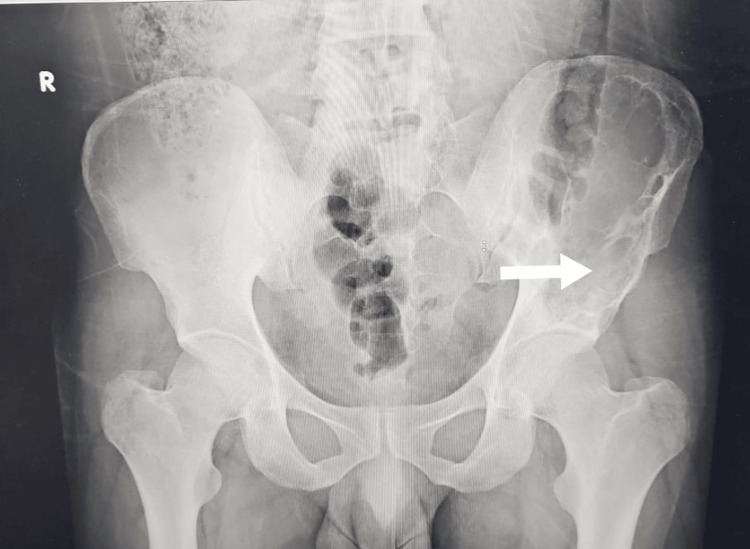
Follow up plain X-ray of pelvis shows the improvement of bone quality in the left iliac bone (arrow)
